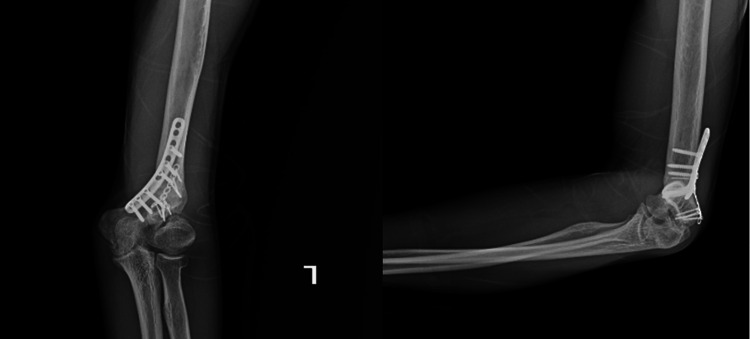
Postoperative radiographic images of the patient taken at nine months
